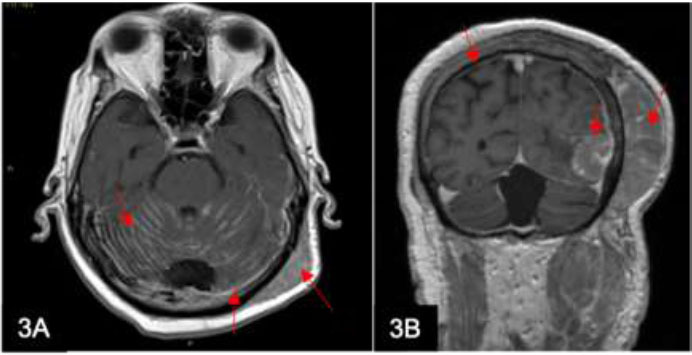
A 63-year-old man with known multiple myeloma presents with a large parieto-occipital scalp mass. Axial T1w post-contrast (A) and coronal T1w post-contrast (B) MRI show diffuse leptomeningeal (arrows) and pachymeningeal enhancement with a large heterogeneous mass involving the left temporal and parietal scalp and bones (arrows). The biopsy and CSF cytology results were positive for myeloma.
